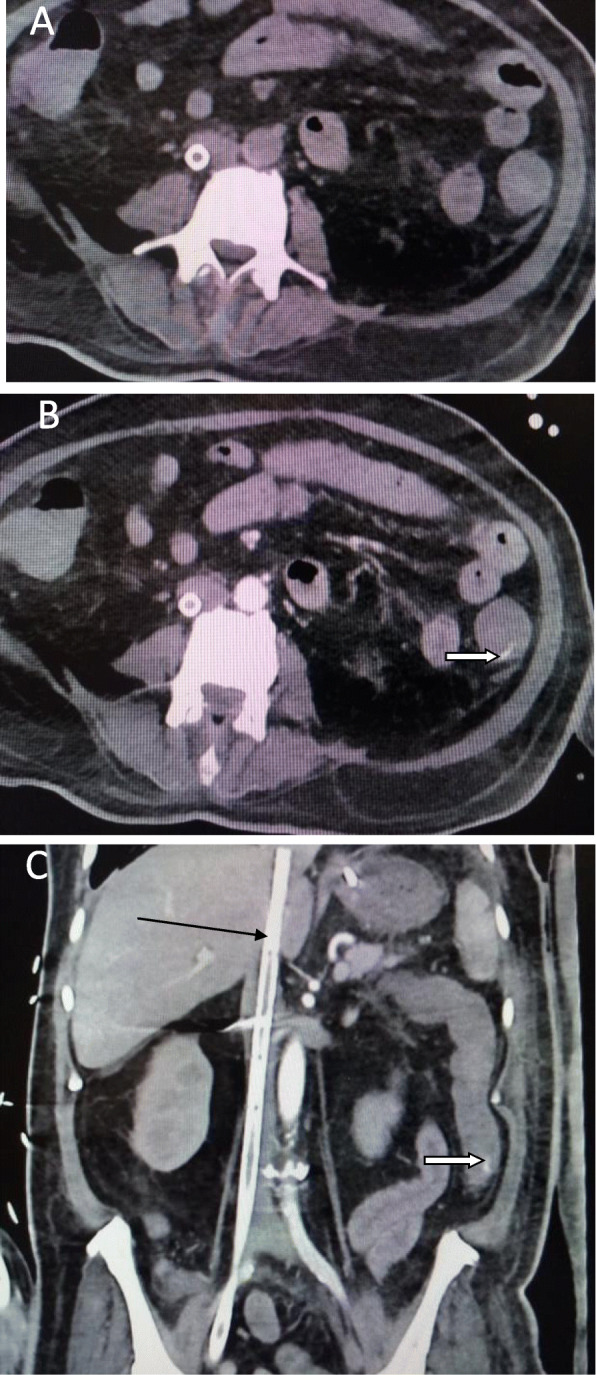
Fifty-six-year-old COVID-19 male patient isolated in the ICU due to respiratory failure with past medical history of DM and HTN then presented with hematochezia. MDCT study was done: a axial non contrast scan of CT of the abdomen and b post contrast axial scans in the arterial phase showing active contrast extravasation in the lumen of the descending colon (open arrow). c Coronal reformatting done showing active contrast extravasation (open arrow), extra corporeal membrane oxygenation device (ECMO) is noted (black arrow)
